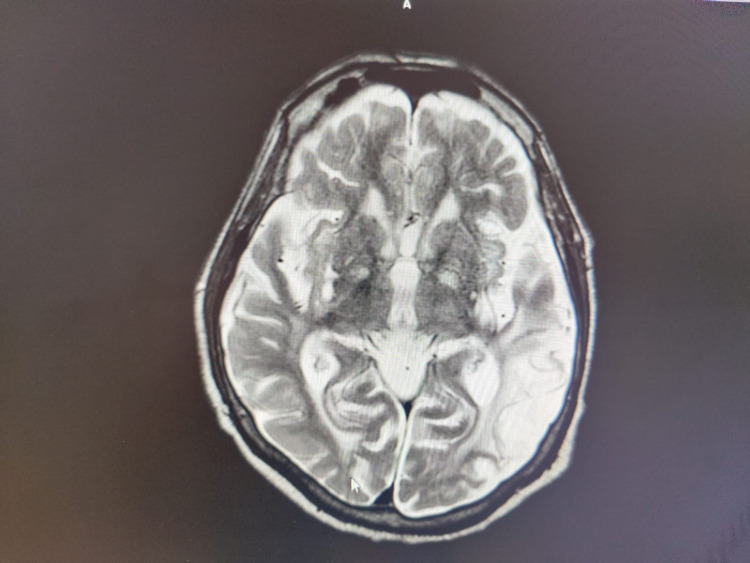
T2W MRI brain plain axial view Gliotic changes and cystic encephalomalacia at left parietooccipital lobes with loss of parenchymal volume. T2W MRI: Transverse relaxation time magnetic resonance imaging
